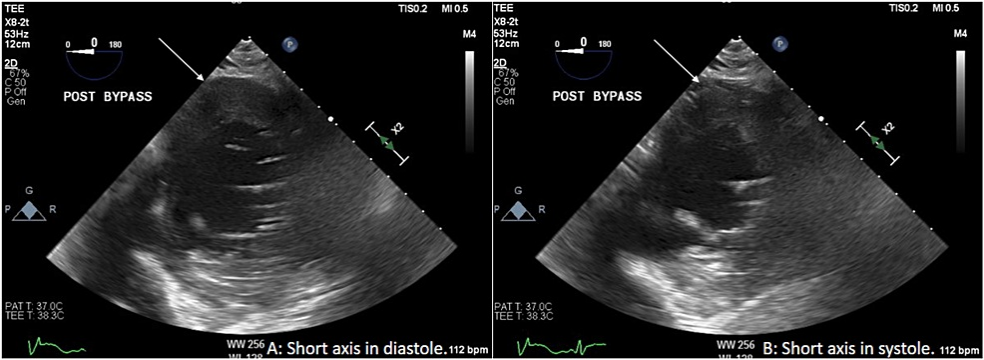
Post cardiopulmonary bypass transesophageal echocardiogram in short axis demonstrating dyskinesis of the inferior wall between diastole (A) and systole (B). White arrows identify an area of dyskinesis.
